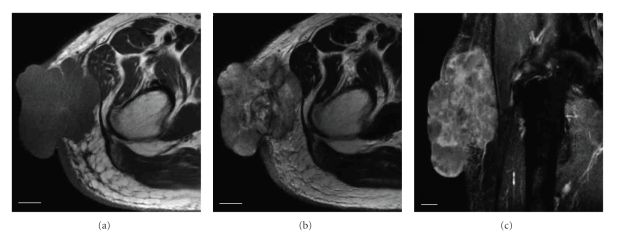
MR images of the tumor in the pre-treatment condition. (a) Axial T1-weighted image. (b) Axial T2-weighted image. (c) Coronal STIR sequence enhanced with Gd-DTPA. Bars = 2 cm. The heterogeneous mass was attached to the fascia lata and expanded across the skin layer.
